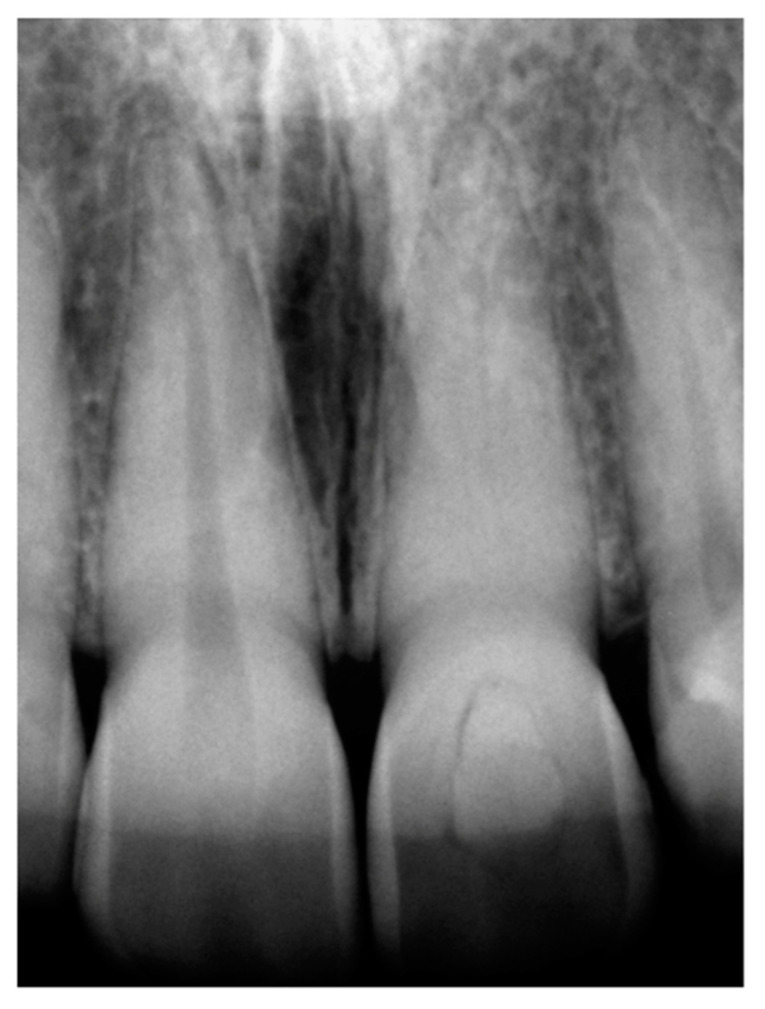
Periapical radiography showing obliteration of the pulp chamber and root canal of the upper left central incisor. Pulp sensitivity test showed positive reaction.
